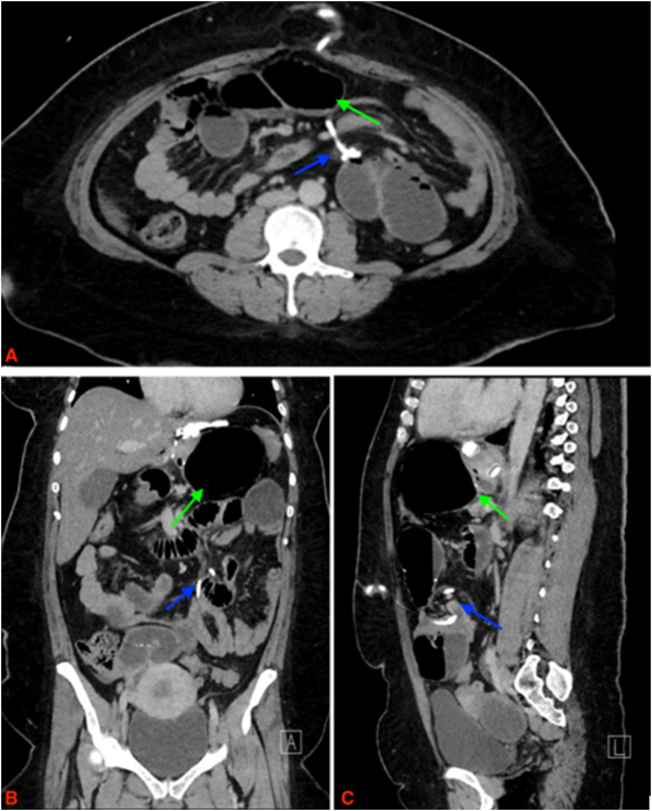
(A) Axial CT scan; (B) sagittal CT scan; (C) coronal CT scan. Imaging of the abdomen and pelvis on admission depicting the coiling of the gastric connecting tube around the transition point of the SBO (blue arrow) and the dilated small bowel segments (green arrow). No closed-loop internal hernia was identified on this scan, which was later seen intraoperatively.
